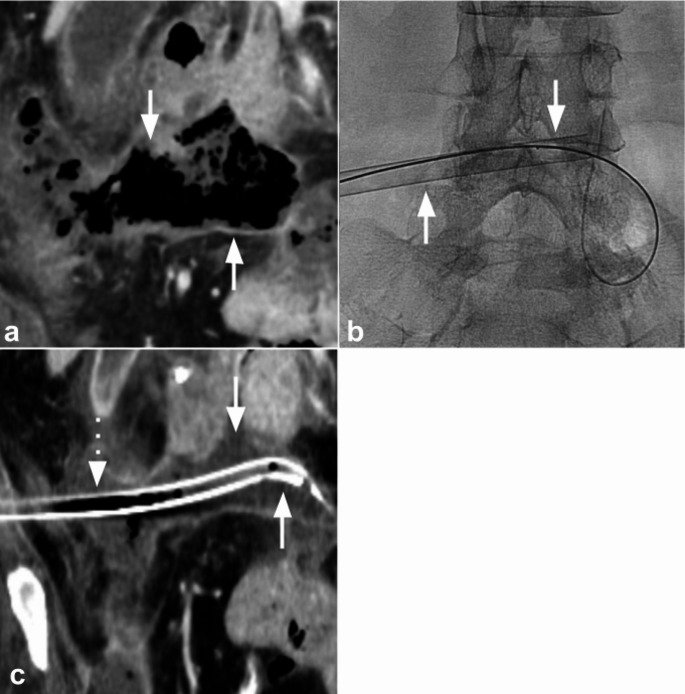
Large-bore aspiration of a walled-off necrotic collection using the FlowTriever Aspiration Catheter in a patient with necrotizing pancreatitis (a) Coronal contrast-enhanced computed tomography (CT) of the abdomen and pelvis shows a 13.2 × 4.5 cm collection consistent with walled-off necrosis (arrows) (b) Fluoroscopic image shows tract dilation and placement of the T24 FlowTriever Aspiration Catheter (arrows) (c) Follow-up contrast-enhanced CT of the abdomen and pelvis obtained four days later shows a reduction in abscess size to 5.0 × 3.8 cm (arrows) with a 24-French drainage catheter in place (dashed arrow)
